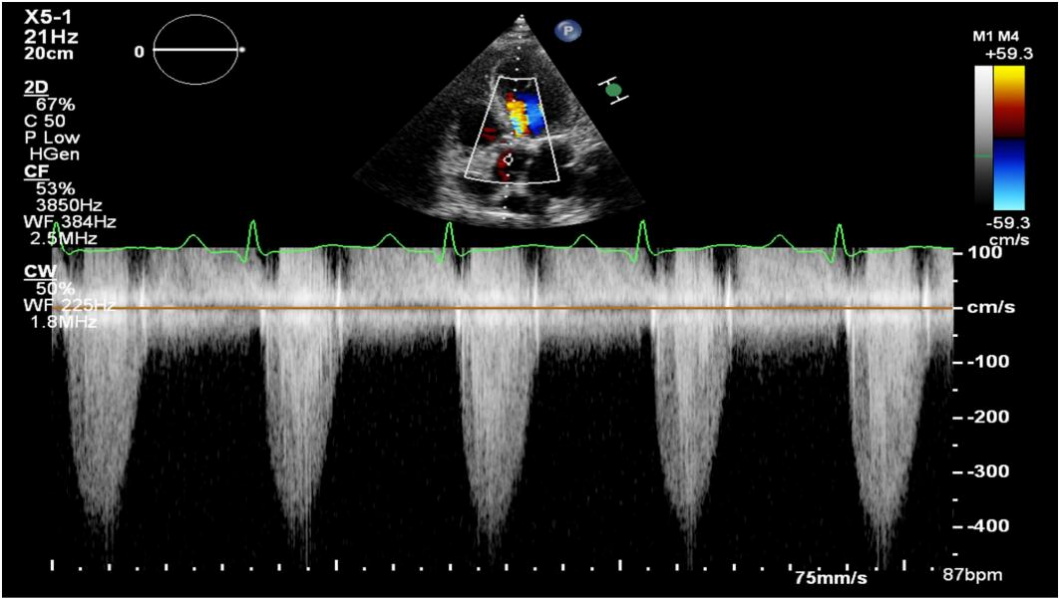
Continuous Doppler on native aortic valve. Continuous Doppler shows an aortic valve peak velocity of 4.31 m/s.
